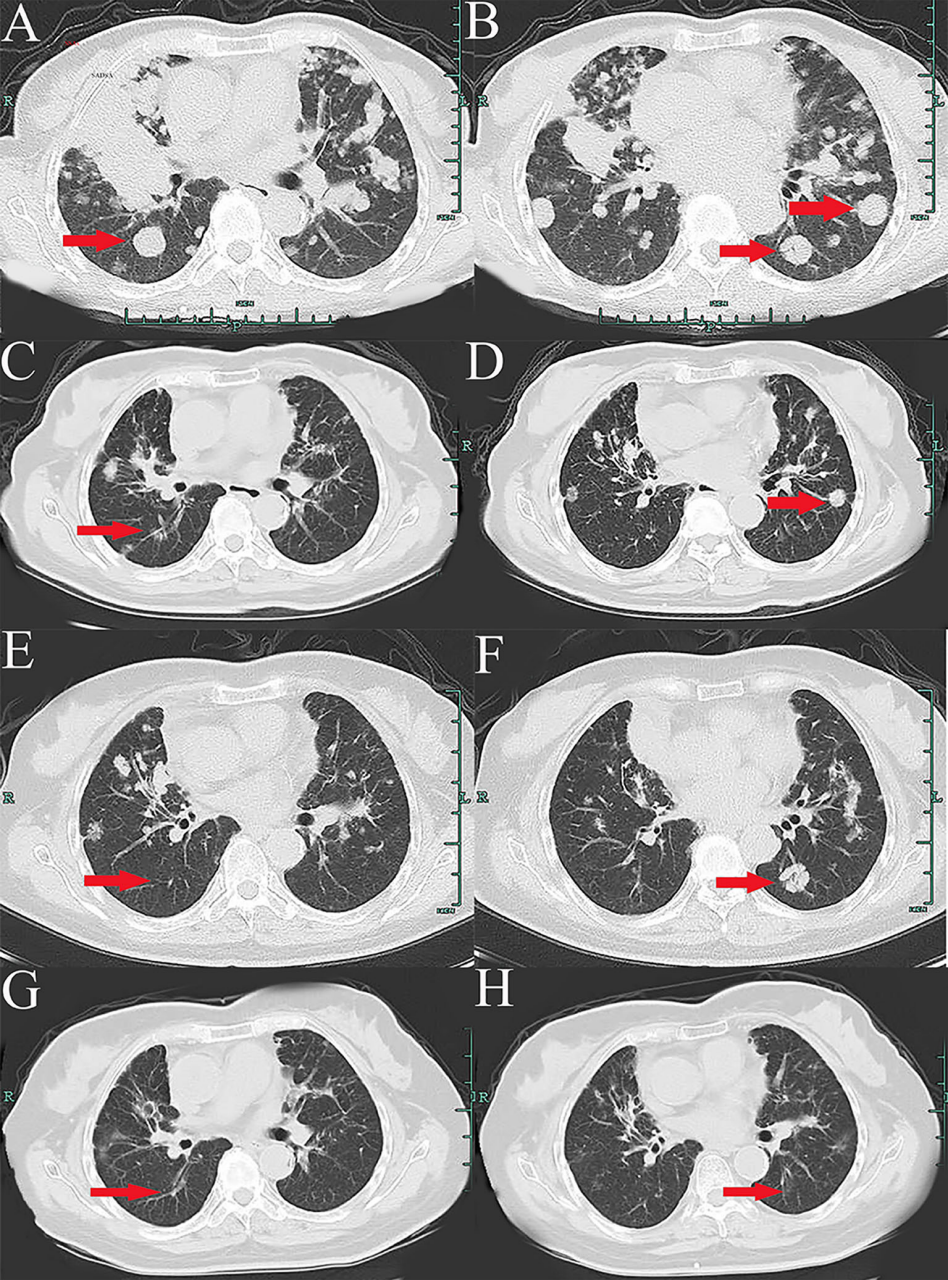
Variations of occupation on mediastinum and both hilar areas by CT images during the treatment (red arrowheads). (A, B), February, 2022. (C, D), March, 2023. (E, F), April, 2024. (G, H), July, 2024.
